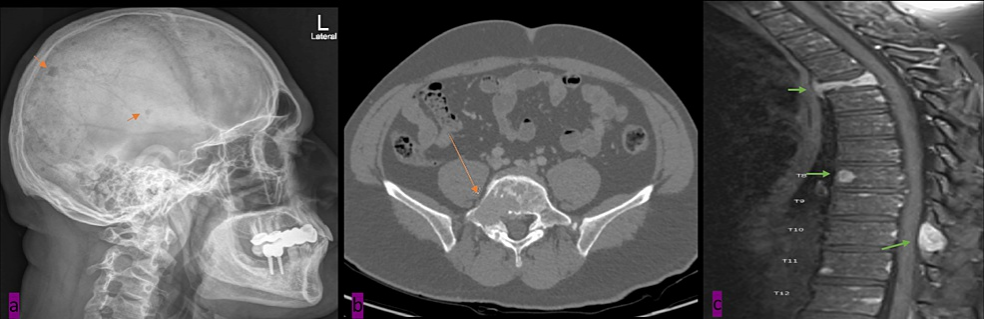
Multiple lytic punch-out bone lesions (Orange arrows) in the skull (a) and right sacral ala (b) multiple thoracic spine lytic lesions show enhancement in MRI T1-FS post-contrast (Green arrows).
